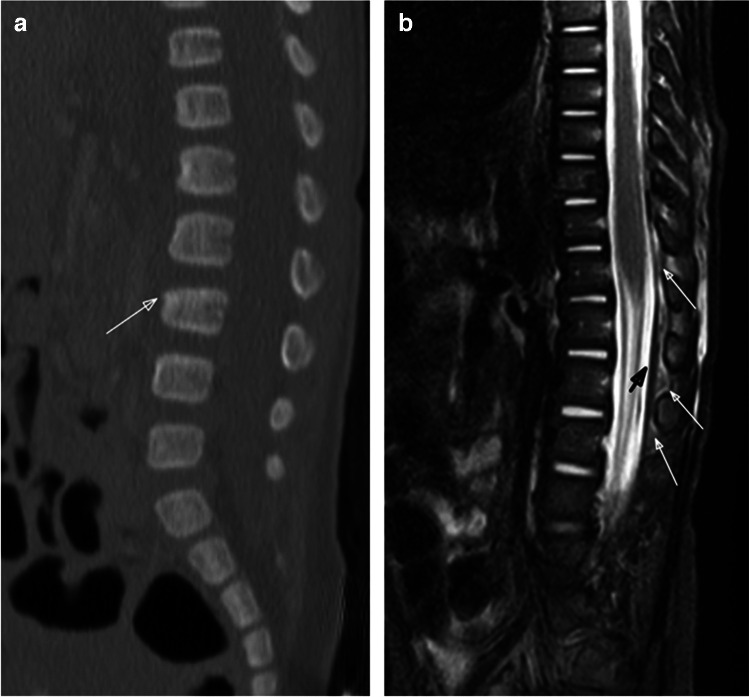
Five-month-old boy presents with bruising. Sagittal bone CT reconstruction of the lumbar spine from an abdominal CT (a) shows anterior wedging of the L3 vertebral body (*arrow*). Sagittal STIR MR image (b) demonstrates epidural fluid/hemorrhage (*white arrows*) and thin subdural hemorrhage (*black arrow*), as well as multilevel interspinous ligament injury/edema and subcutaneous soft tissue edema. No associated marrow edema corresponding to the L3 vertebral body wedging
